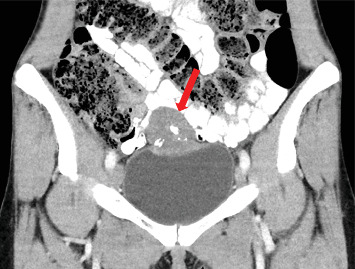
CT of the abdomen in the sagittal plane, showing the tumorous mass on the urinary bladder dome with the presence of calcifications (red arrow).
